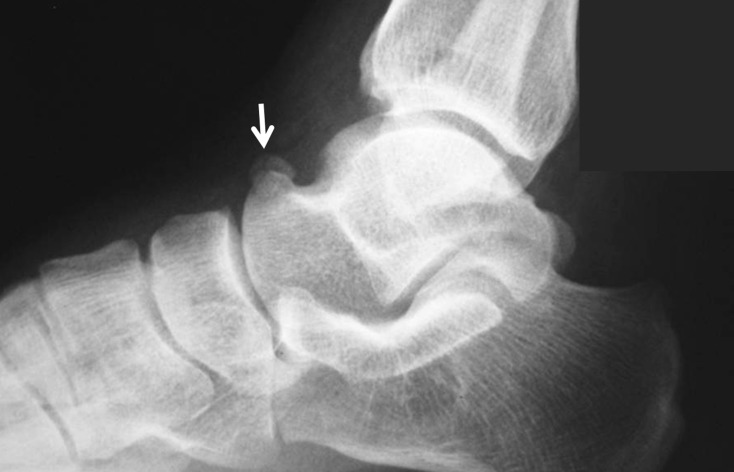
Radiographic appearance of the os supratalare located over the head of the talus (white arrow)
